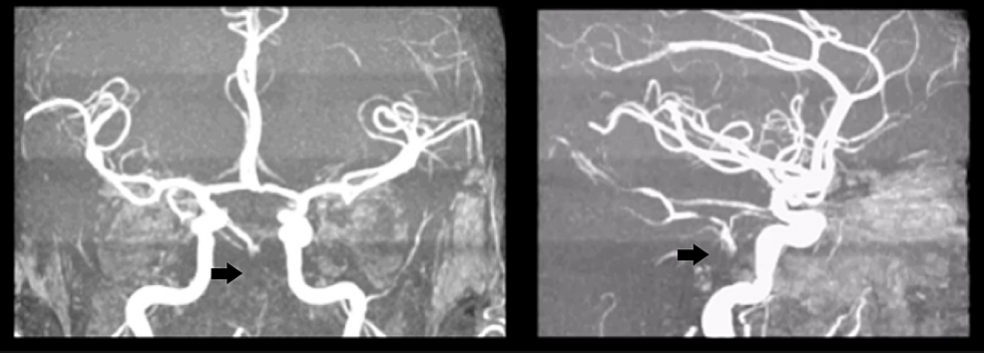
Angio-MRI with no flux in basilar artery. MRI, magnetic resonance imaging.
